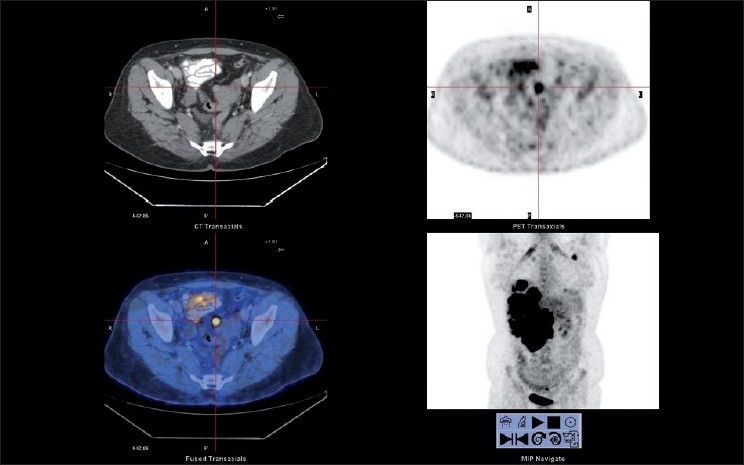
Transaxial view of CT, PET and fused images showing focal FDG avid uterine lesion
